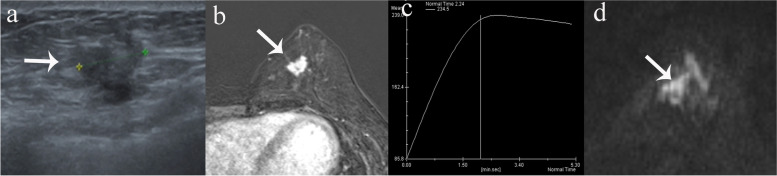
True-positive MRI finding for a lesion with US BI-RADS 4B category. a Gray-scale US revealed 14 mm irregular hypoechoic mass with indistinct margin on the left breast and 4B category was given. b, c Dynamic contrast-enhanced imaging showed an irregular mass with irregular margin and plateau kinetics. d The lesions displayed hyperintensity on DWI, with mean ADC values of 0.72 × 10^–3^ mm^2^/s. BI-RADS 5 category was given on MRI. Pathology showed high-grade ductal carcinoma in situ
